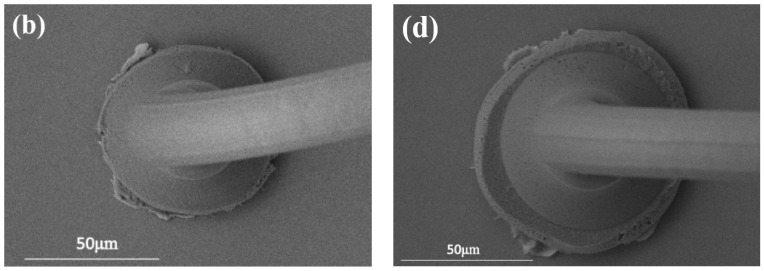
Morphology of Pd120 ball-bonded points under different bonding pressures/ultrasonic power ((a) 50 g/120 mW; (b) 50 g/110 mW; (c) 50 g/130 mW; (d) 55 g/120 mW).
